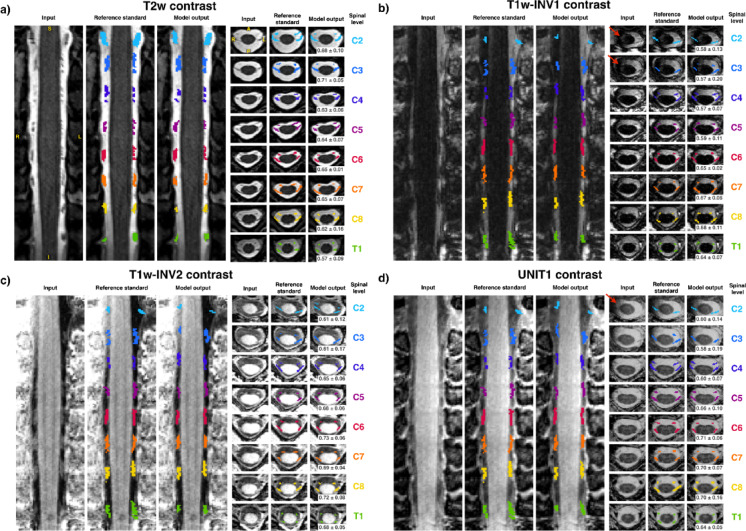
Coronal and axial views of representative rootlet segmentations. (a) Segmentations on a T2w MRI scan. (b) Segmentations on a T1w-INV1 MRI scan. (c) Segmentations on a T1w-INV2 MRI scan. (d) Segmentations on a UNIT1 MRI scan. The red arrows point to an artifact possibly caused by B1 + inhomogeneities. Rows represent individual rootlet levels from C2 to T1. Numbers represent the mean ± SD Dice score across participants for each spinal level compared to the reference standard labels.
